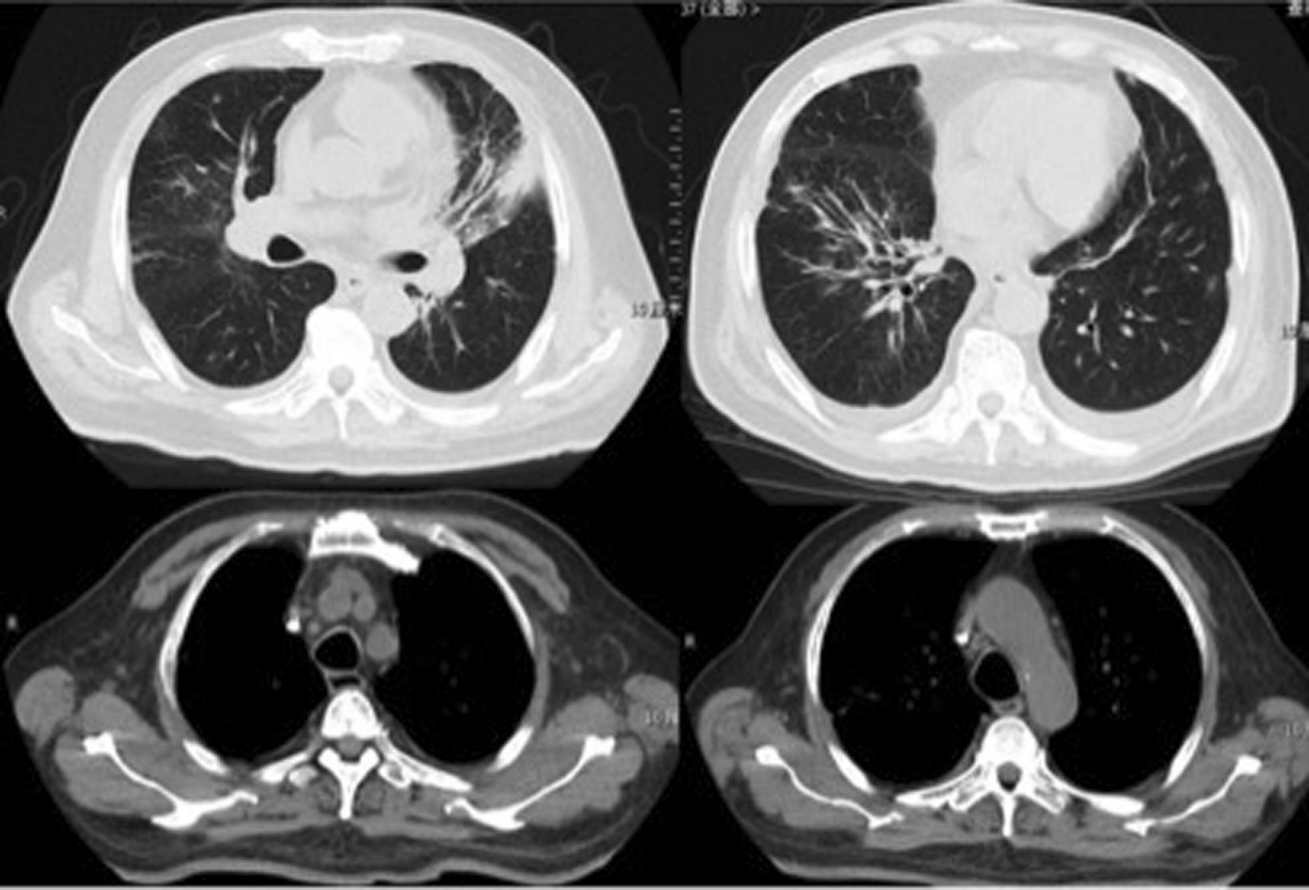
Chest CT scan on day 50 of hospitalization: lesions in both lungs were obviously absorbed, and the enlarged lymph nodes in the mediastinum and hilum were reduced or disappeared
